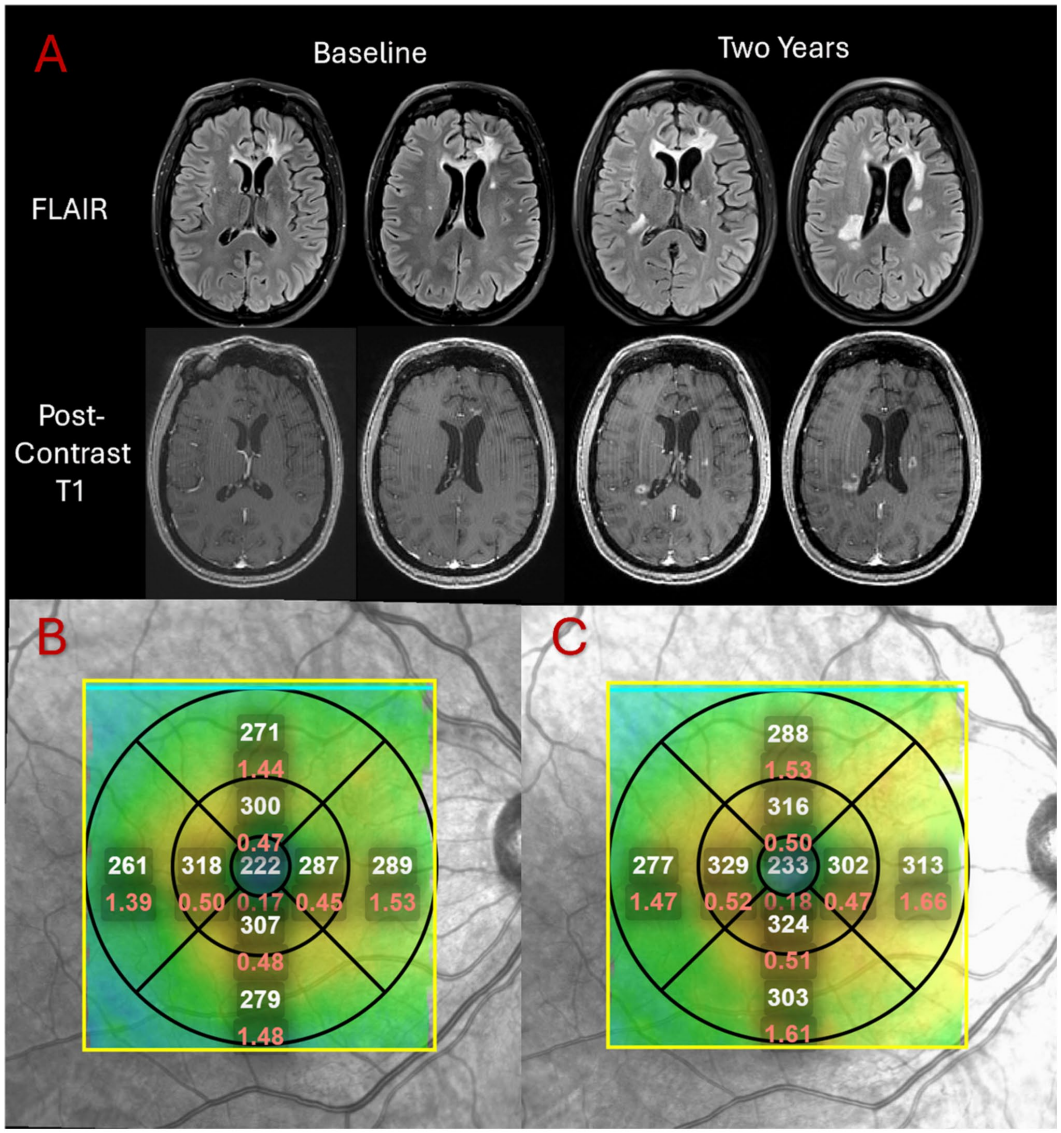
Example of macular volume, central subfield thickness, and white matter hyperintensities in a single participant. (A) Figure depicting FLAIR and post-contrast T1-weighted brain images from one participant at baseline and 2-year follow-up. Extension of existing lesions and new lesions can be noted. (B) Figure depicting an optical coherence tomography image of the macula in the same participant at baseline. The Early Treatment of Diabetic Retinopathy Study (ETDRS) grid is centered around the fovea, with average thickness values of each segment presented in white and volume measures in mm^3^ presented in red. (C) Figure depicting a macular optical coherence tomography image of the same participant at end-of-study showing lower TMV values at follow-up, quantified by adding volume measurements in all segments.
